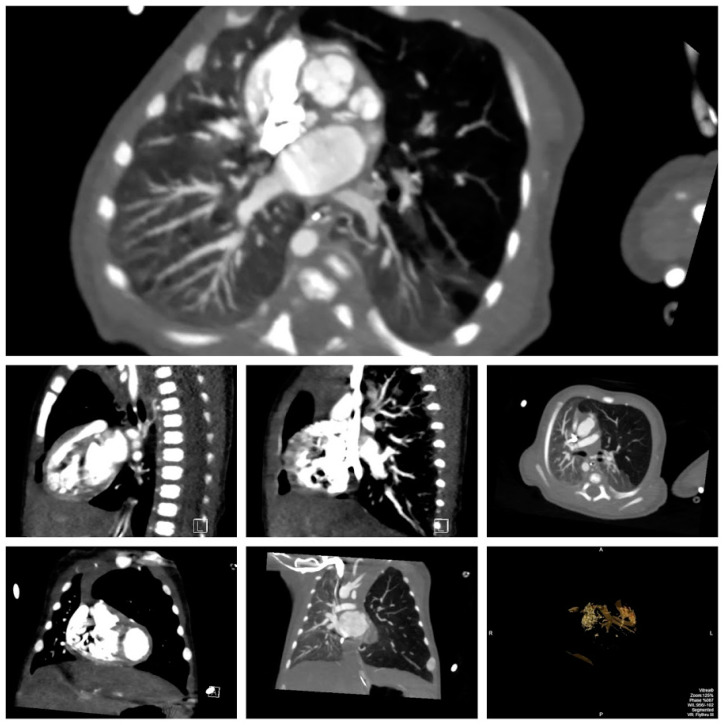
Multiple views on cardiac computed tomography (CT) demonstrating tertiary tracheal bronchus supplying the lingula with stenosis at the origin, double outlet right ventricle (DORV) with transposition of the great vessels, hyperinflated left upper lobe, partially atelectatic left upper and lower lobes, herniation to the right, and 3D reconstruction of airway.
